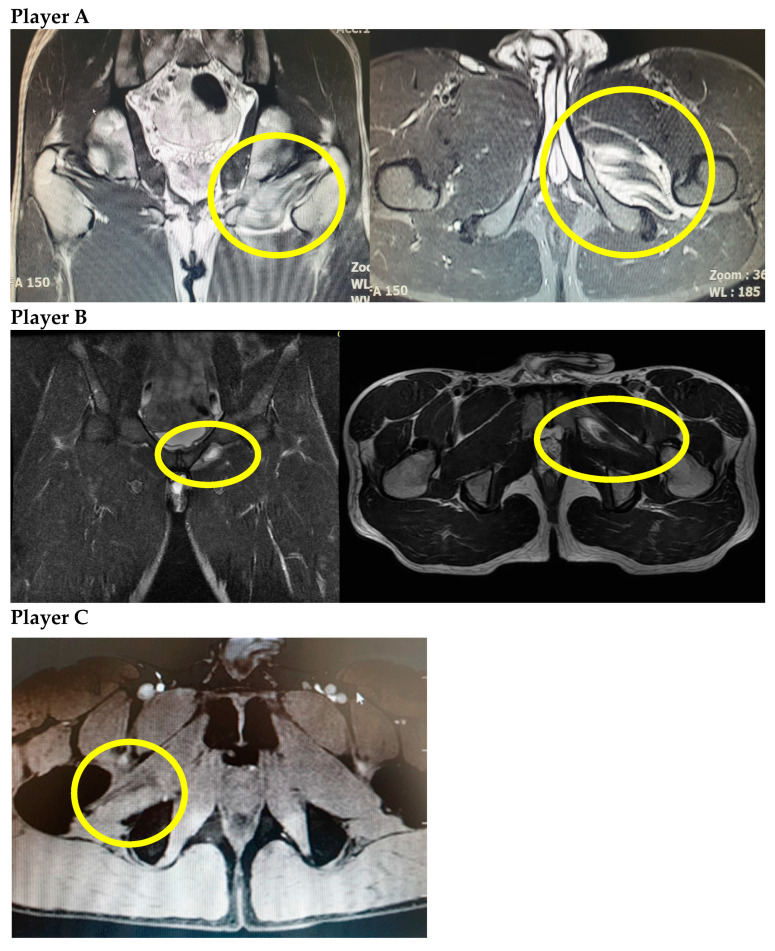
MRI of obturator externus injury in three professional soccer players. Player A: Hip joint MRI demonstrates grade II injury of left obturator externus muscle, coronal image (left) and axial image (right). Player B: Hip joint MRI demonstrates grade II injury of left obturator externus muscle, coronal image (left) and axial image (right). Player C: Hip joint MRI demonstrates grade I injury of right obturator externus muscle.
